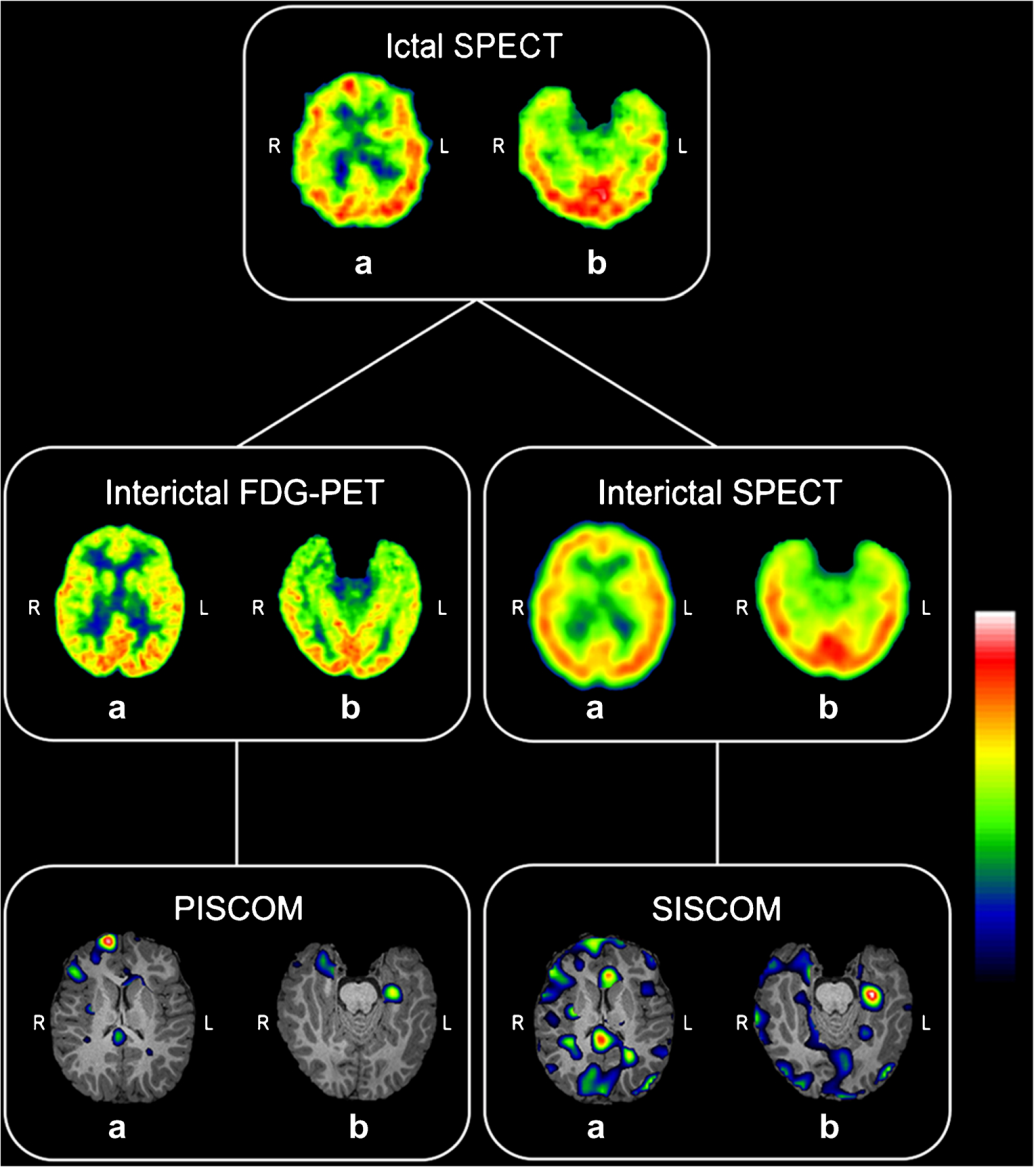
Example patient (P22, a 4-year-old child with a confirmed right frontal epileptogenic zone) showing discordant PISCOM and SISCOM results. Axial ictal and interictal SPECT, original FDG PET, PISCOM and SISCOM images of the brain at the frontal (*a*) and temporal (*b*) lobes. The PISCOM images show successful identification of the SOZ in the right frontal lobule (*a*) while the left medial temporal lobe shows focal uptake with lower activity interpreted as propagation (*b*). The SISCOM images show diffuse uptake in right frontal lobe interpreted as indeterminate activity (*a*), and focal activity in the left medial temporal lobe incorrectly identified as the SOZ (*b*). *Colour scale* intensity values in the SPECT and PET images, relative differences between ictal and interictal image values in the PISCOM and SISCOM images. All images are coregistered with MRI images. *L* left, *R* right
